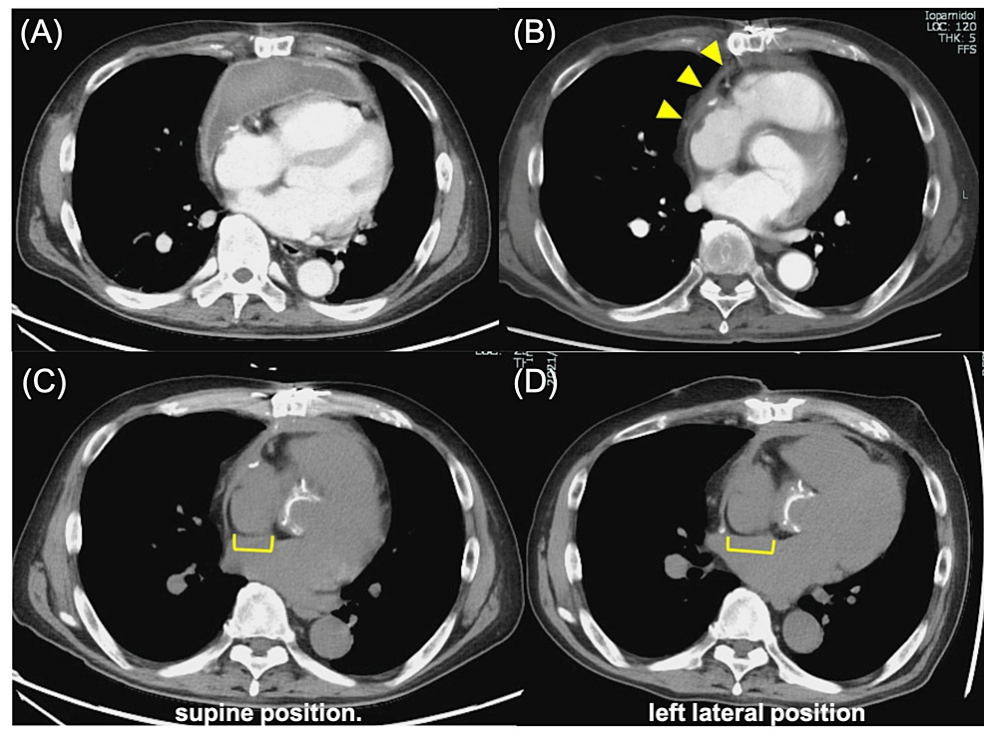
Chest computed tomography after the Bentall procedure. (A) A few days after the Bentall procedure, a pericardial hematoma was observed. (B) After drainage of the pericardial hematoma, pleural thickening was observed as indicated by the yellow arrow. (C) Image taken before patent foramen ovale closure in the supine position. (D) Image taken with the patient in the left lateral position. The atrial septum was stretched more than in the supine position.
